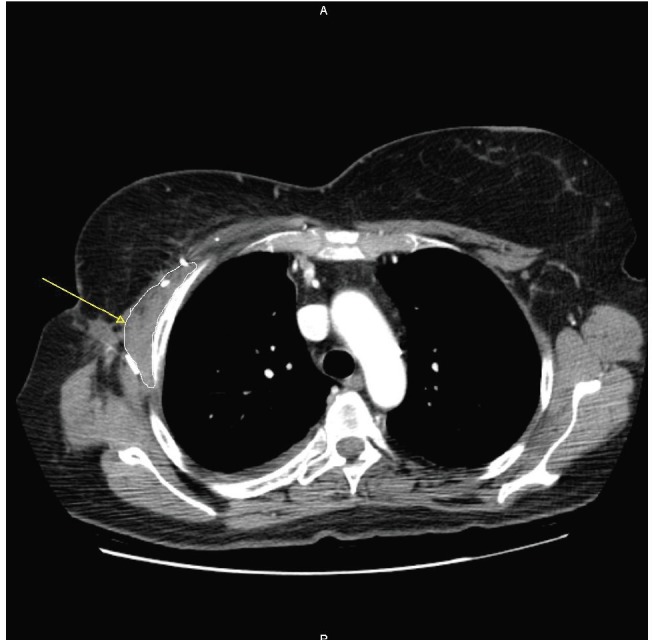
An axial CT scan, after 12 months of treatment, showing an initial downsizing of the lesion, contoured by a white line.
